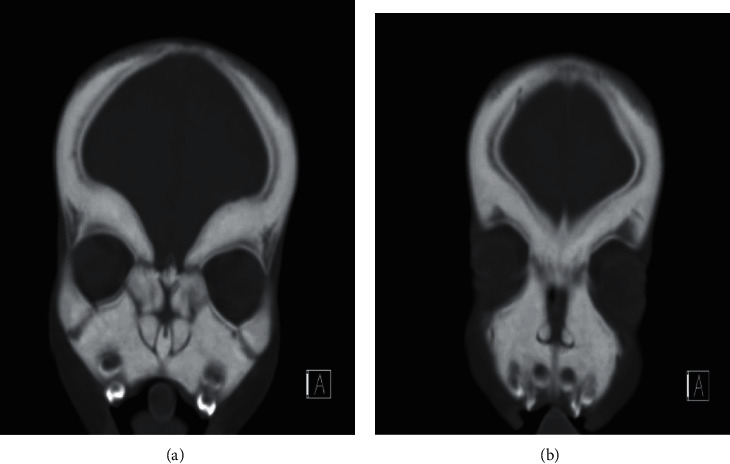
The CT scan of the brain shows extensive thickening of the facial bone.
